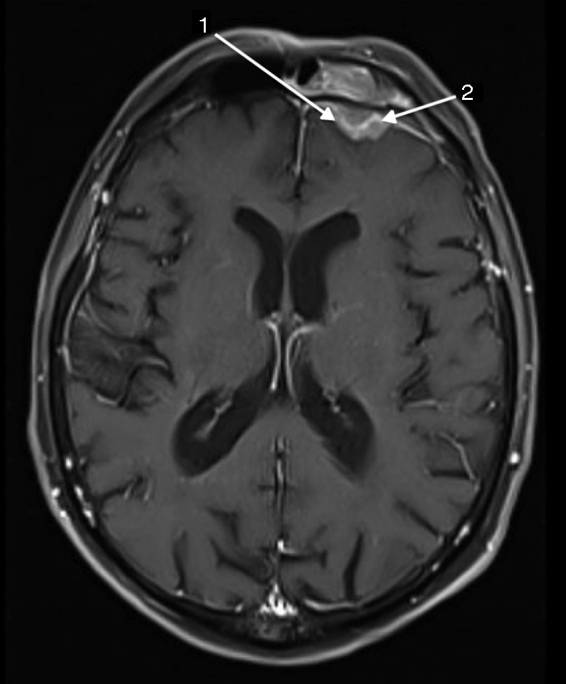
The sinus frontal lesion (MRI, T1). We observed *a* ≥ 2 mm dural thickening (1) and nodular dural enhancement (2) demonstrating the dural invasion.
